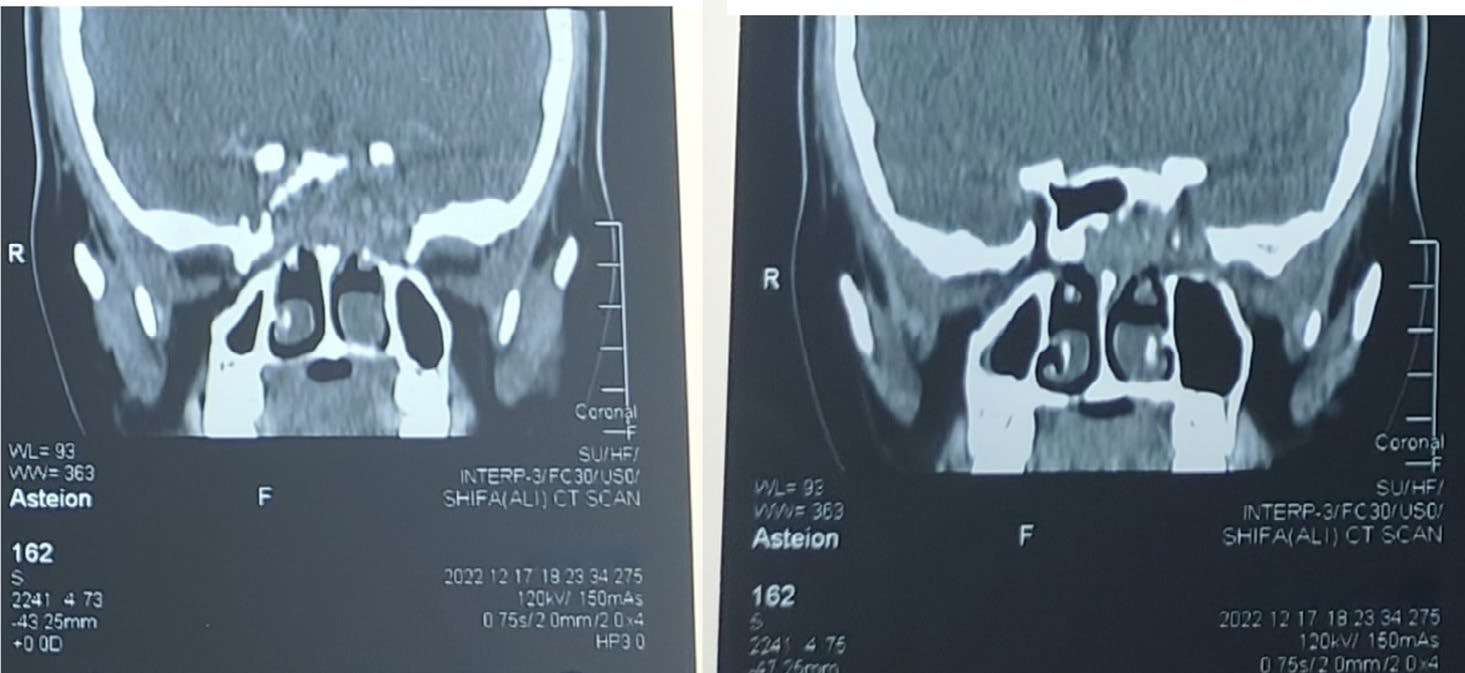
Coronal computed tomography: Bony erosion surrounding sphenoid sinus mass.
